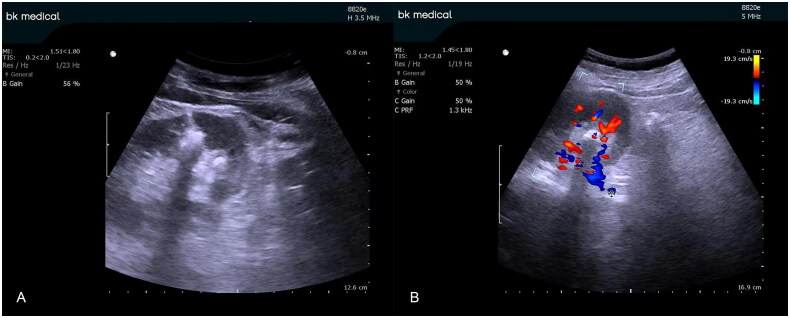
B-ultrasound image of the solitary kidney during percutaneous renal puncture (A). color Doppler ultrasonography image of the same solitary kidney after percutaneous renal puncture (B). Red indicates the artery; blue indicates veins.
